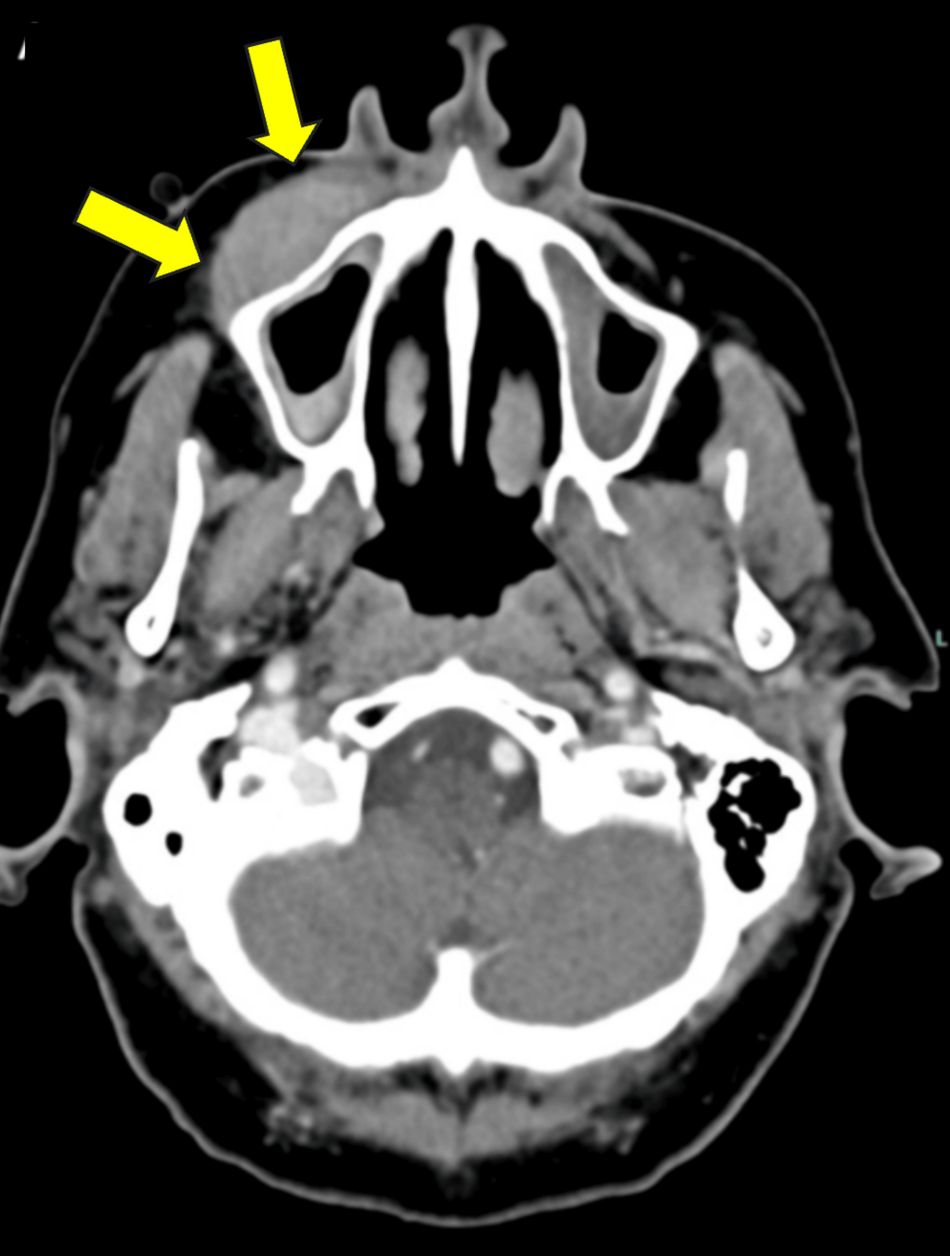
Coronal computed tomography scan with contrast of the right facial mass prior to surgical treatment. There is a 3.3 by 1.2 cm soft tissue mass abutting the anterior wall of the right maxillary sinus. No underlying osseous destruction is seen. The soft tissue of the nasopharynx, orbits, and brain is unremarkable.
